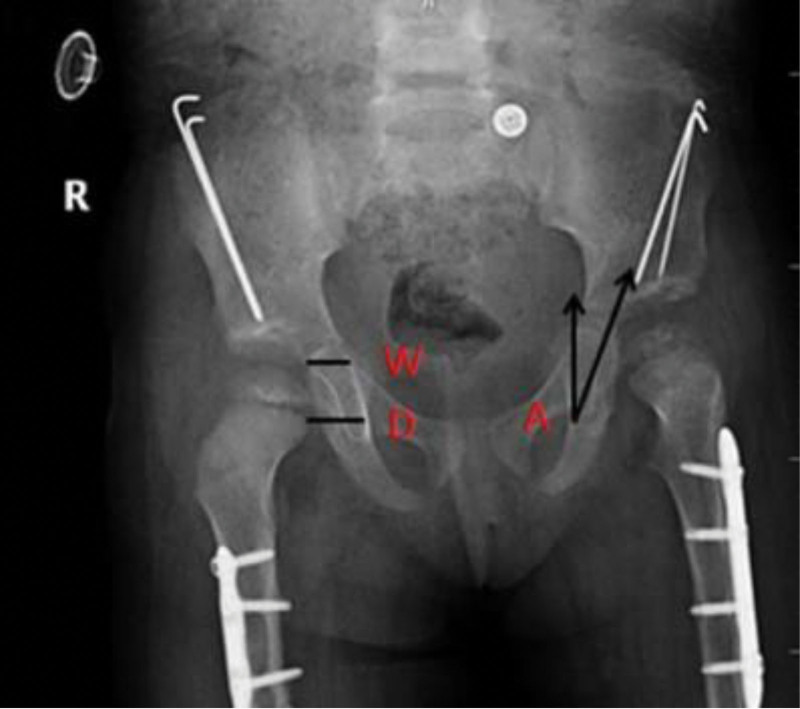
Smith c/b ratio measurement. Pelvic radiograph of a 26-month-old girl who underwent salter innominate osteotomy of her right hip. Measurement of Smith c/b ratio.
